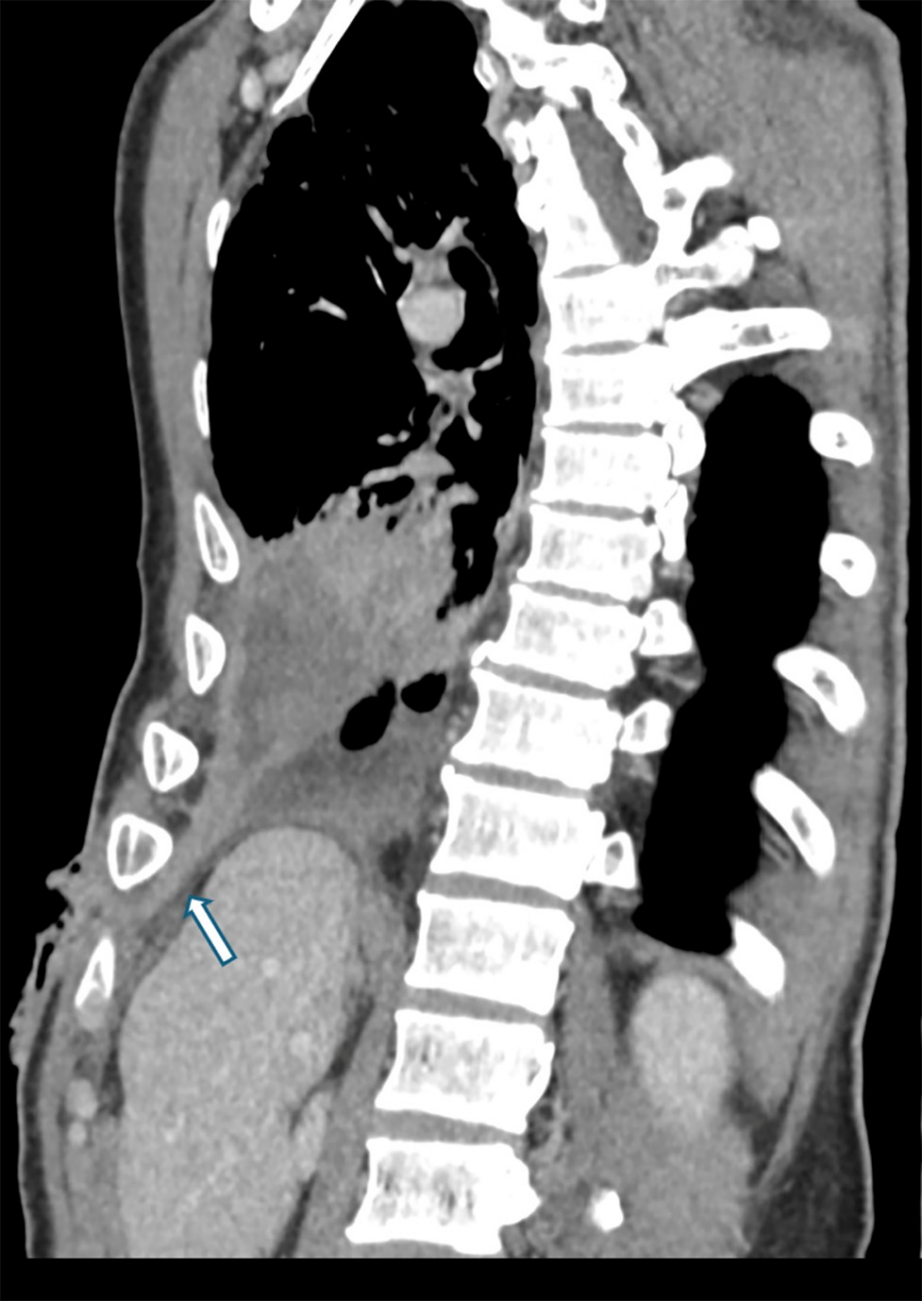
Sagittal CT demonstrating hypodense tract between pleural cavity and skin (arrow).
